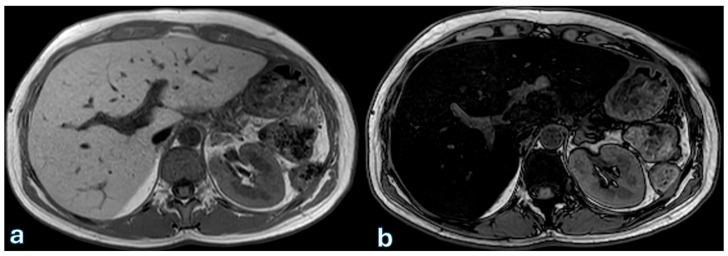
MR T1-weighted image sequences acquired in-phase (a) and out-of-phase (b) showing a marked signal drop between the in-phase and out-of-phase sequences, consistent with diffuse hepatic steatosis. This finding was observed in a patient at the first follow-up, conducted one month after surgery for pancreatic adenocarcinoma located in the head.
